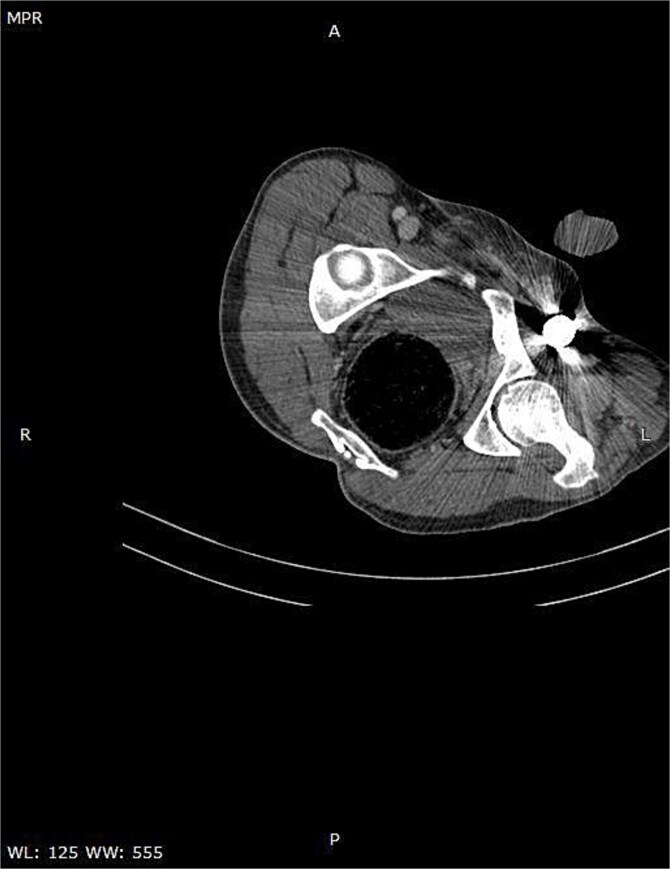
Axial CT image demonstrating the metallic rod positioned in close proximity to the femoral sheath without evidence of vascular injury.
